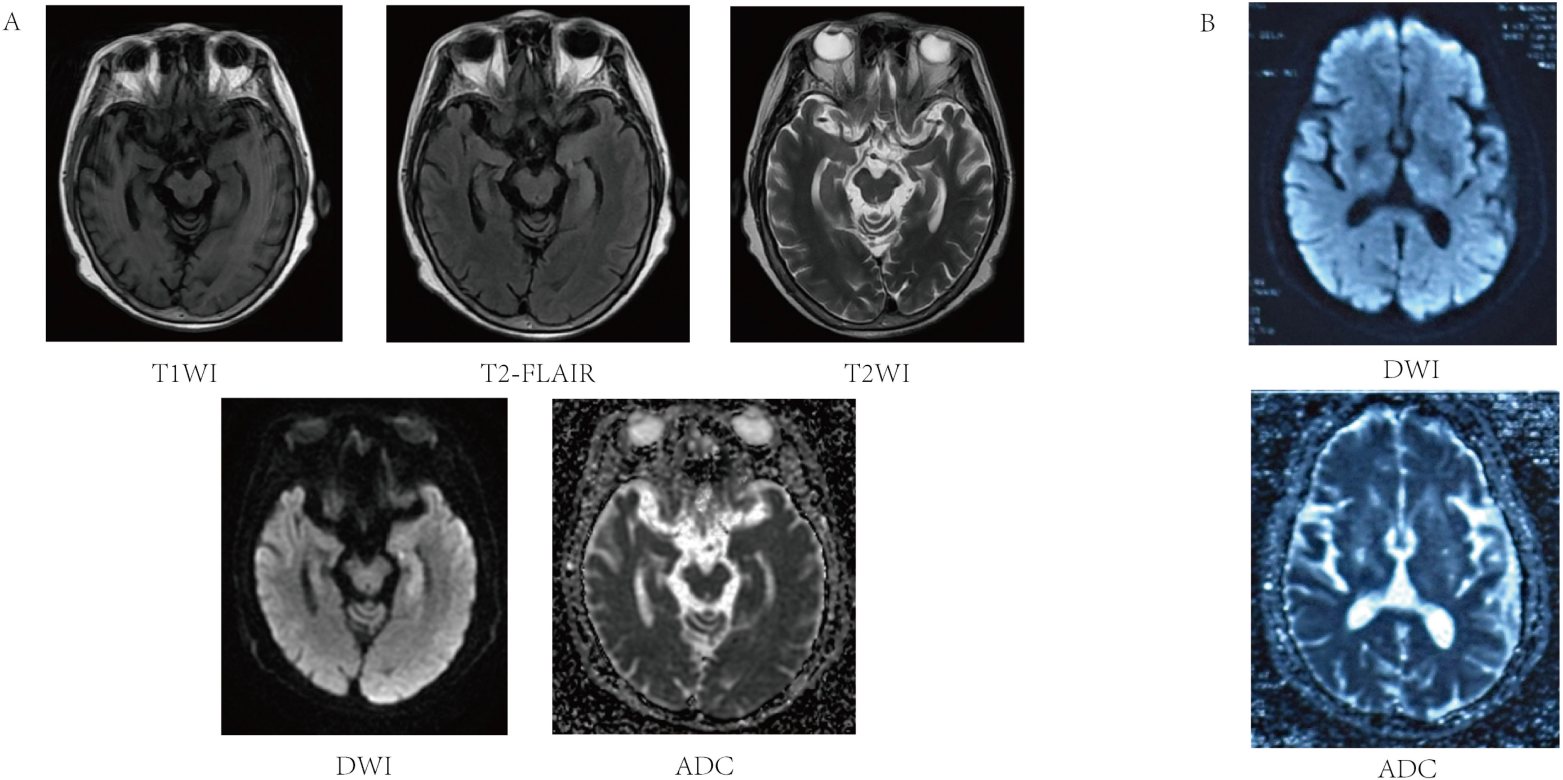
Magnetic resonance image of the patient’s acute cerebral infarction and left hippocampal abnormality. (A) T1 left hippocampus with reduced signal in T1WI sequence, slightly higher signal in T2-FLAIR, slightly higher signal in T2WI sequence increased signal in DWI sequence, and iso-signal on ADC maps. (B) Acute ischemic lesion in the left thalamus showing characteristic DWI hyperintensity and ADC hypointensity.
